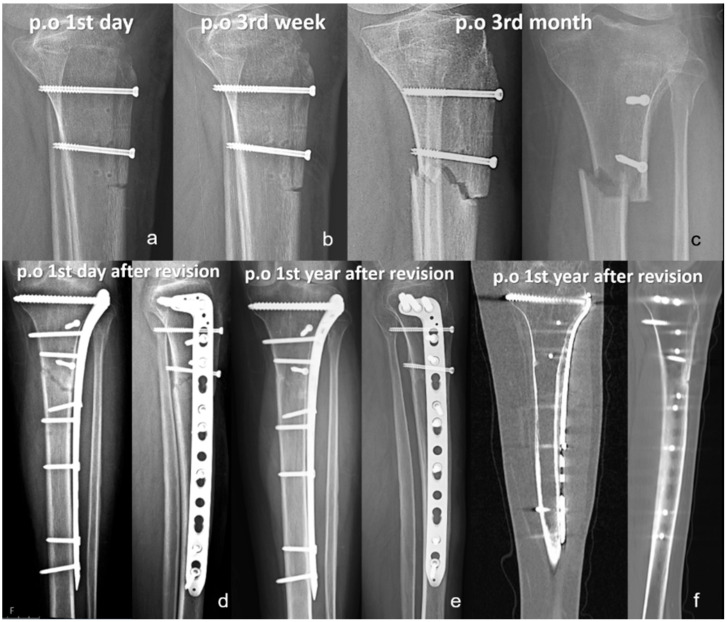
Radiographic follow-up of the patient who developed a tibial fracture after tibial tubercle osteotomy (TTO) and underwent successful revision with plate fixation. (a) Postoperative day 1 radiograph showing proper alignment and fixation. (b) Third-week radiograph showing maintained stable fixation of the osteotomy. (c) Third-month radiograph demonstrating a displaced transverse tibial fracture originating from the distal end of the osteotomy site. (d) Immediate postoperative radiographs following open reduction and internal fixation using a proximal tibial locking plate. (e) One-year follow-up anteroposterior and lateral radiographs demonstrating complete fracture healing and stable fixation. (f) One-year postoperative CT scan confirming cortical continuity and radiological union at both the osteotomy and fracture sites.
